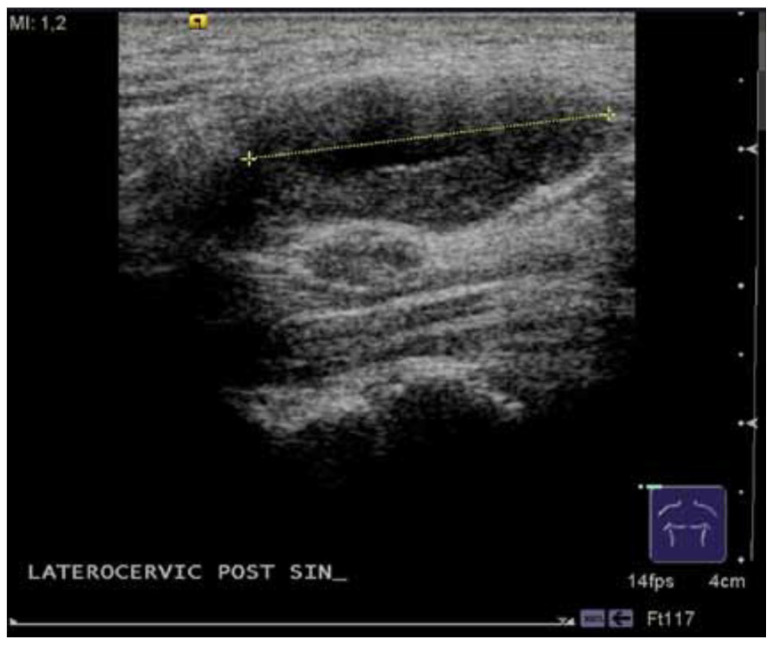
Ultrasonography of a vagal schwannoma.
